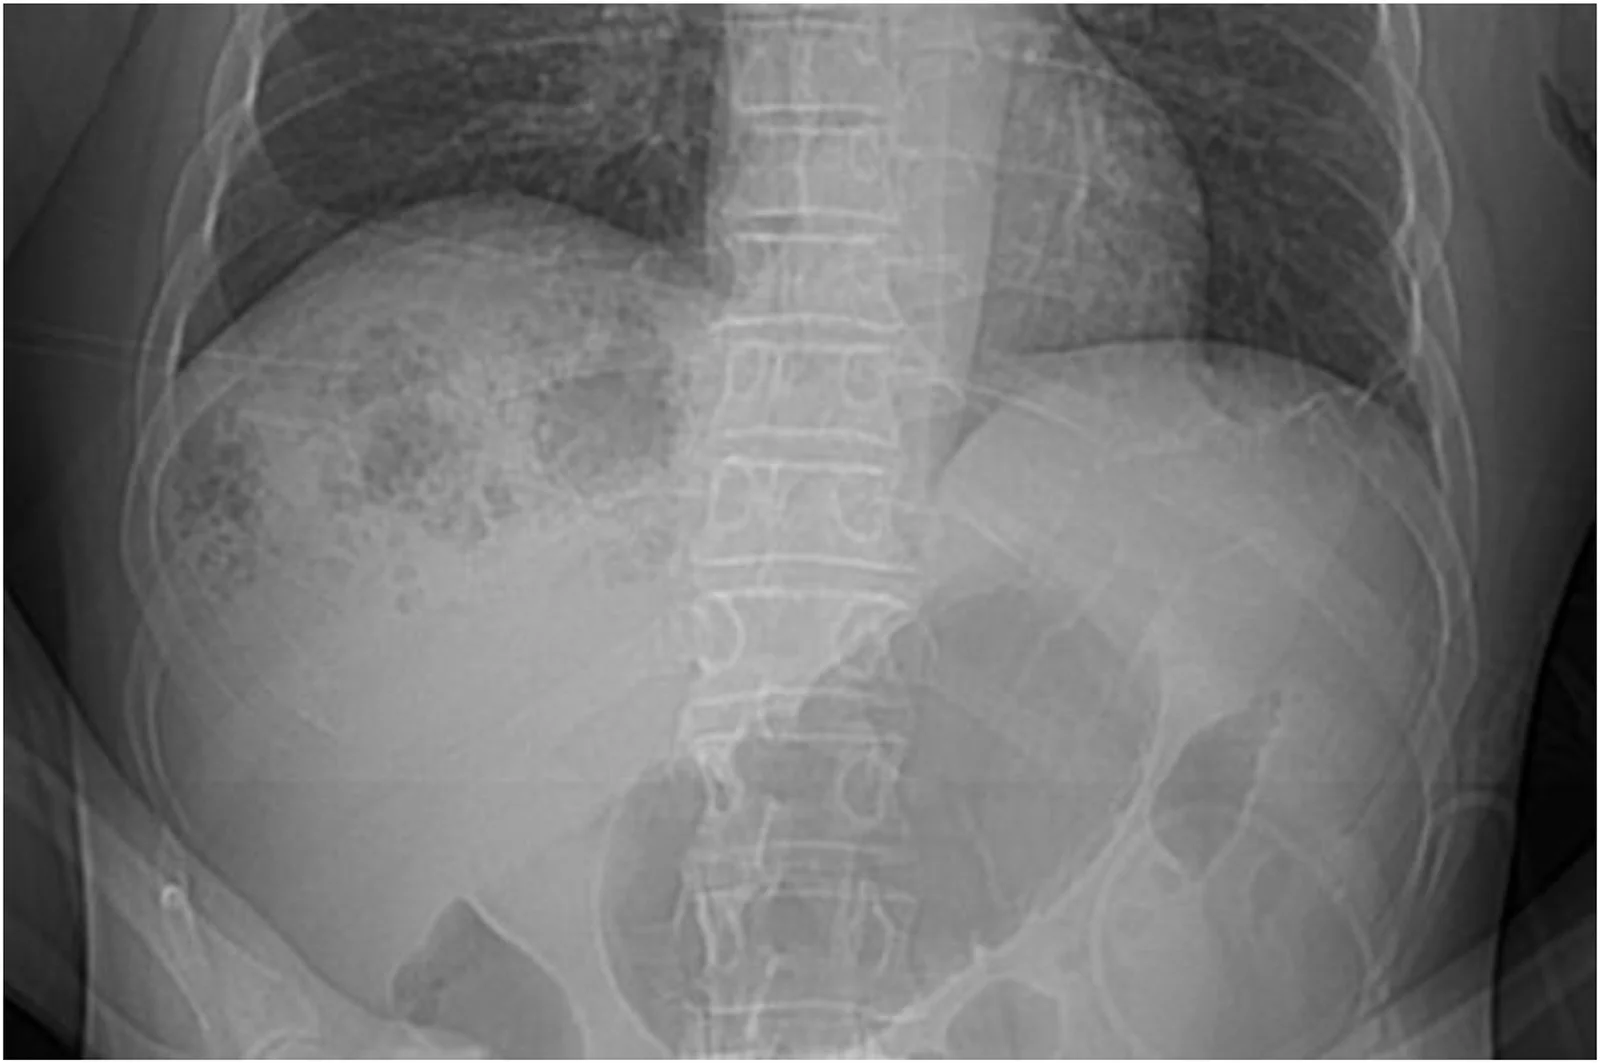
Coronal scout view from the CT scan. There are patchy areas of gas density in the right upper quadrant projected over the liver.
